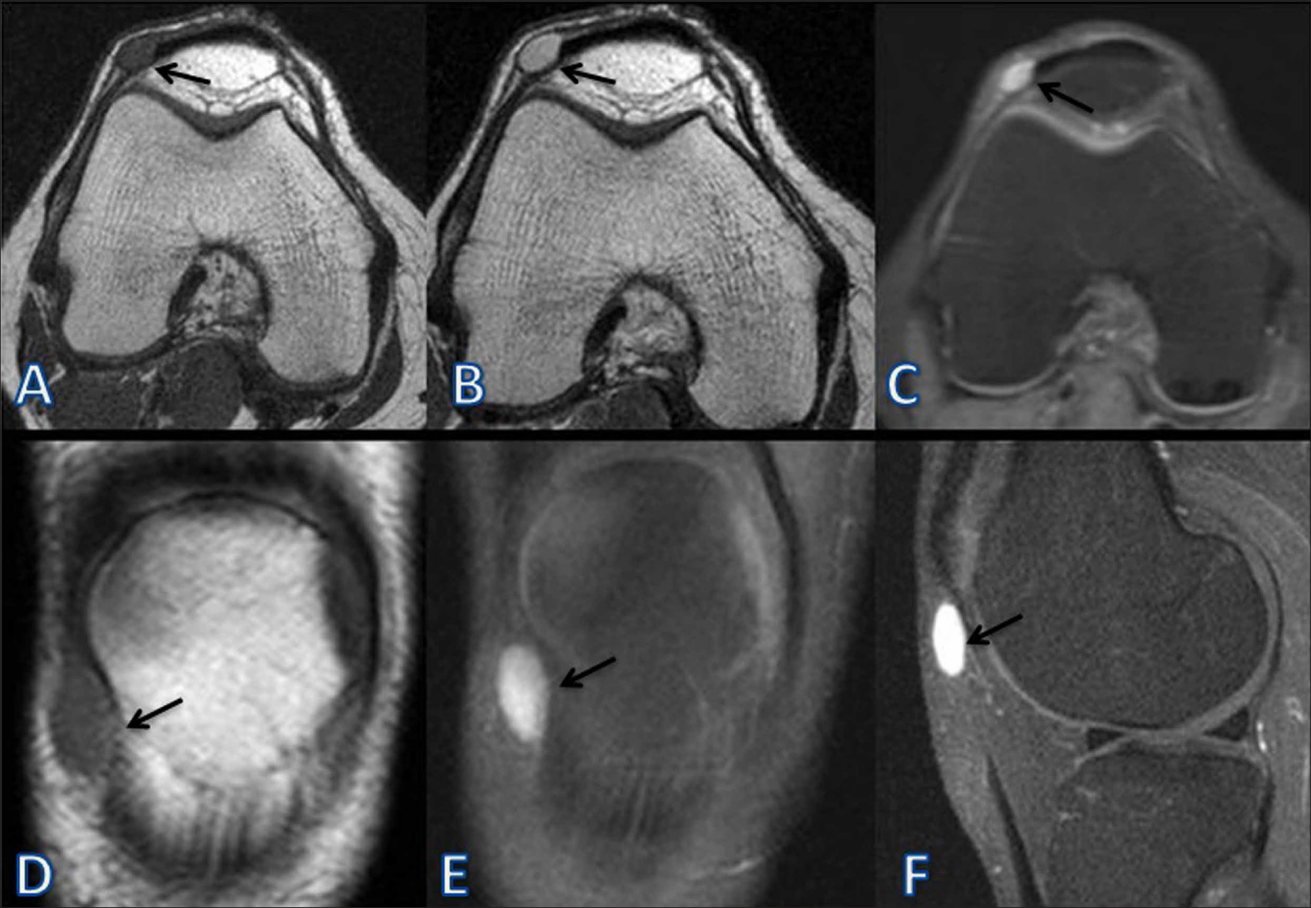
This is a preoperative MRI of the right knee. Axial (A) and coronal (D) T1-weighted pre-contrast images show well-circumscribed, hypointense soft tissue lesion adjacent to the lateral patellar retinaculum. The axial PD-weighted imaging with fat suppression (C) image shows a well-circumscribed, marked homogenous hyperintense subcutaneus lesion. Post-contrast axial (B), fat-supressed coronal (E), and sagital (F) T1-weighted images show prominent enhancement of the tumor and a sharp outline.
